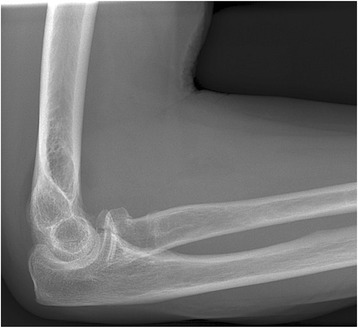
associated injury (coronoid fracture) on radiograph
